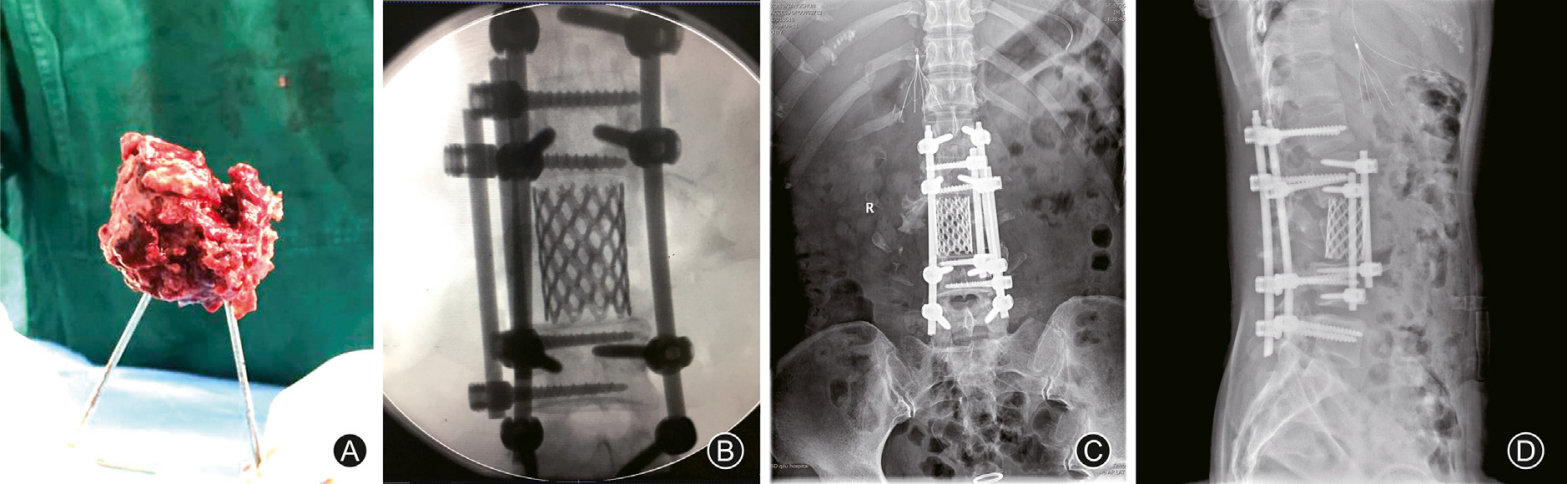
Second anterior internal fixation. We performed vertectomy of the fractured L_3_ vertebrae body (A) and placed a cage between L_2_ and L_4_ (B). Morselized bone harvested from L_3_ was packed into cage. The spinal alignment was restored as implied by postoperative radiography plain of coronal (C) and sagittal (D).
